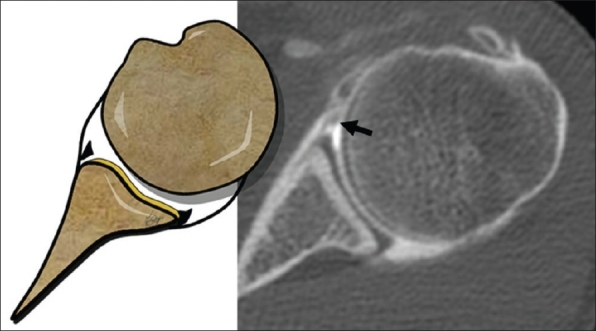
Axial CT-Arthrography image of a 26-year-old man shows non-displaced Bankart lesion, otherwise known as Perthes lesion (*arrow*)
